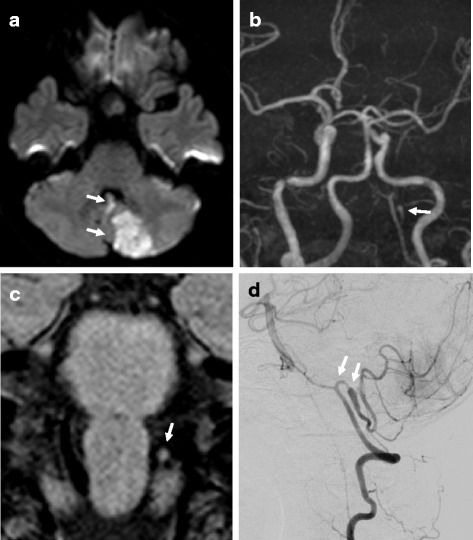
Imaging findings in Case 2. MRI at 4 days after admission showed high-intensity signal areas in the cerebellar vermis and the left cerebellar hemisphere on DWI (a, arrow), the loss of the proximal portion of the left PICA and dilatation of the adjacent distal portion on MRA (b, arrow), and a high-intensity signal area at the dilatation portion on T1-VISTA (c, arrow). A left vertebral angiogram at 8 days after admission showed the “pearl and string sign” at the proximal portion of the left PICA (d, arrow)
